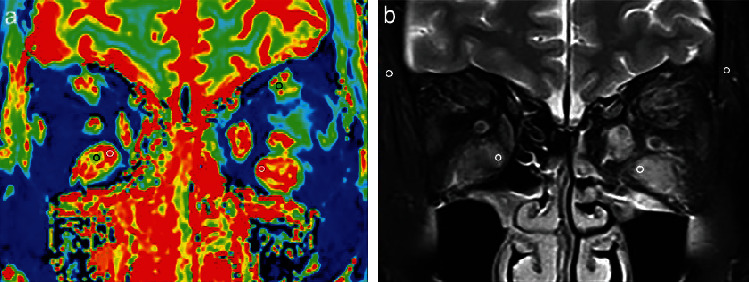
Methods for measurements of T1RT_HS_, T1RT_CS_, and SIR: coronal T1 mapping (a) and coronal fat-suppressed T2-weighted imaging (b) in a 52-year-old male with active TAO. Two circular regions of interest measuring 5–10 mm^2^ were placed in the area with the highest (white) and lowest (black) signal intensity by naked eye, representing T1RT_HS_ and T1RT_CS_, respectively (a). Meanwhile, two similar-sized regions of interest were manually placed in the area of the most inflamed EOM with the highest signal intensity as well as ipsilateral temporal muscle (b). T1RT_HS_, T1RT_CS_, and SIR values of the left/right eye were 2426/2006 ms, 1365/1422 ms, and 4.14/3.95, respectively. T1RT = T1 relaxation time; HS = hot spot; CS = cold spot; SIR = signal intensity ratio; TAO = thyroid-associated ophthalmopathy.
